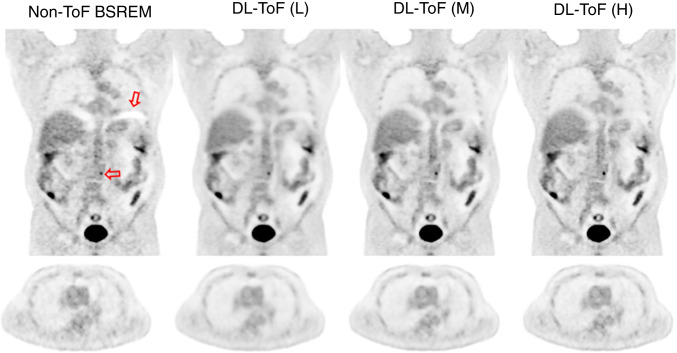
DL-ToF enhancement of a representative test subject with a BMI of 26.0 kg/m^2^ and weight of 84.8 kg with an injected activity of 160 MBq scanned on a GE Discovery IQ non-ToF PET/CT scanner (slice thickness 3.8 mm). The arrow shows attenuation artefacts and a small lesion in vertebra. Display window: 0–5 SUV
